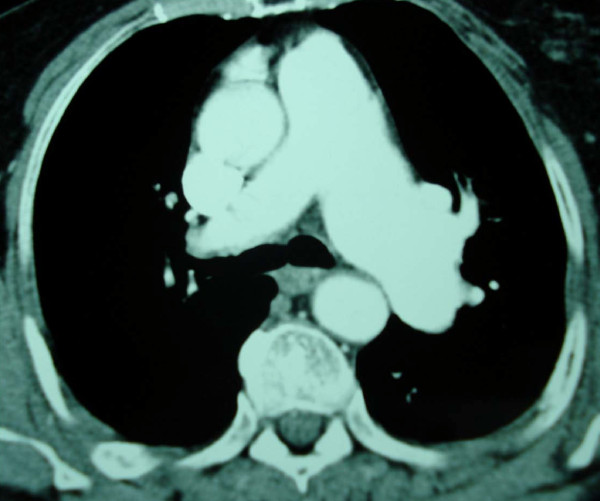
Chest spiral CT angiography of the second patient (Case 2) reveals an aneurysmal dilation of the pulmonary trunk and the left pulmonary artery.
